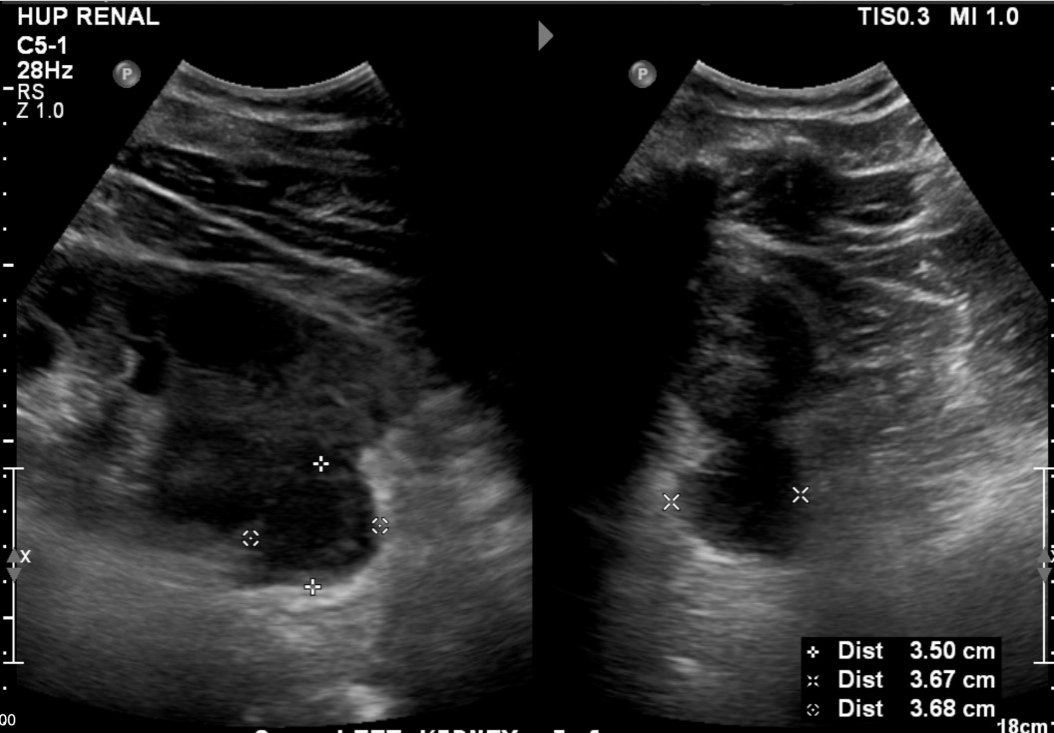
Figure 3. Referral kidney ultrasound of left kidney in long axis demonstrating suspected plasmacytomas 3.7 cm in maximum diameter at the inferior pole. Left: left kidney long axis. Right: left kidney short axis.
